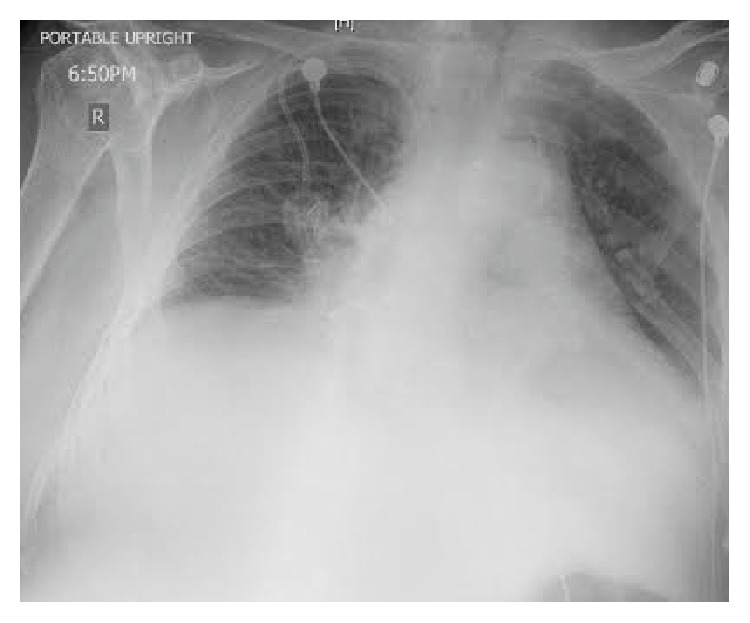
Chest X-ray suggestive of an enlarged cardiac silhouette.
